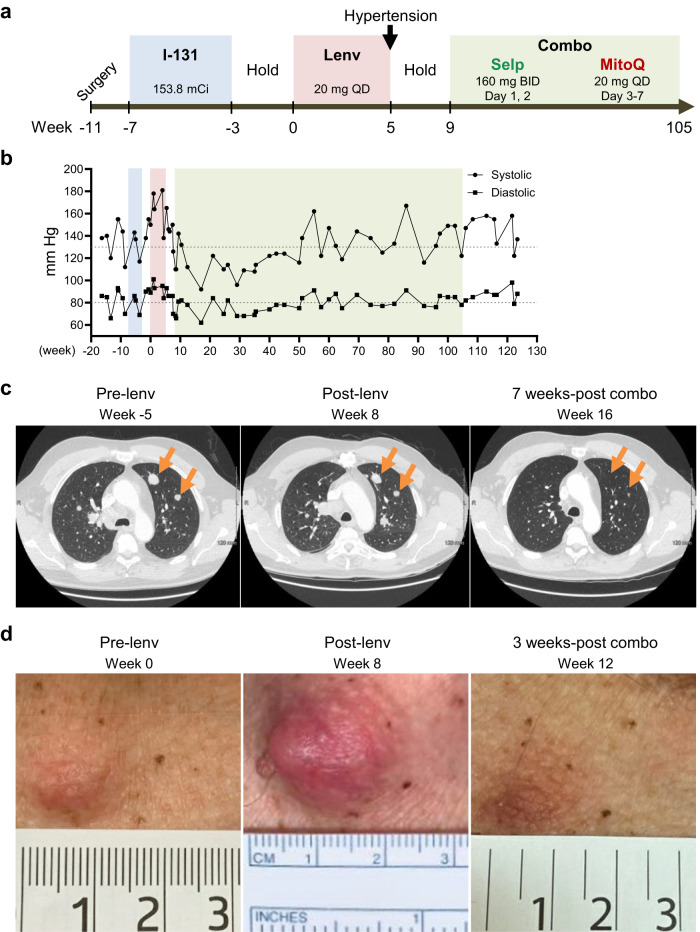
Treatment of the patient with *CCDC6-RET* fusion metastatic PTC. a Treatment timeline. b Blood pressure changes during the treatment. Dotted line indicates normal range of blood pressure. c CT scans of lung metastasis (orange arrows) prior to lenvatinib treatment (left), after stopping lenvatinib (middle), and after receiving 7 weeks of the selpercatinib and MitoQ combination therapy (right). Colors in b indicate the same treatment schedule shown in a. d Images of chest wall skin metastasis prior to lenvatinib treatment (left), after stopping lenvatinib (middle), and after receiving 3 weeks of the combination therapy (right).
